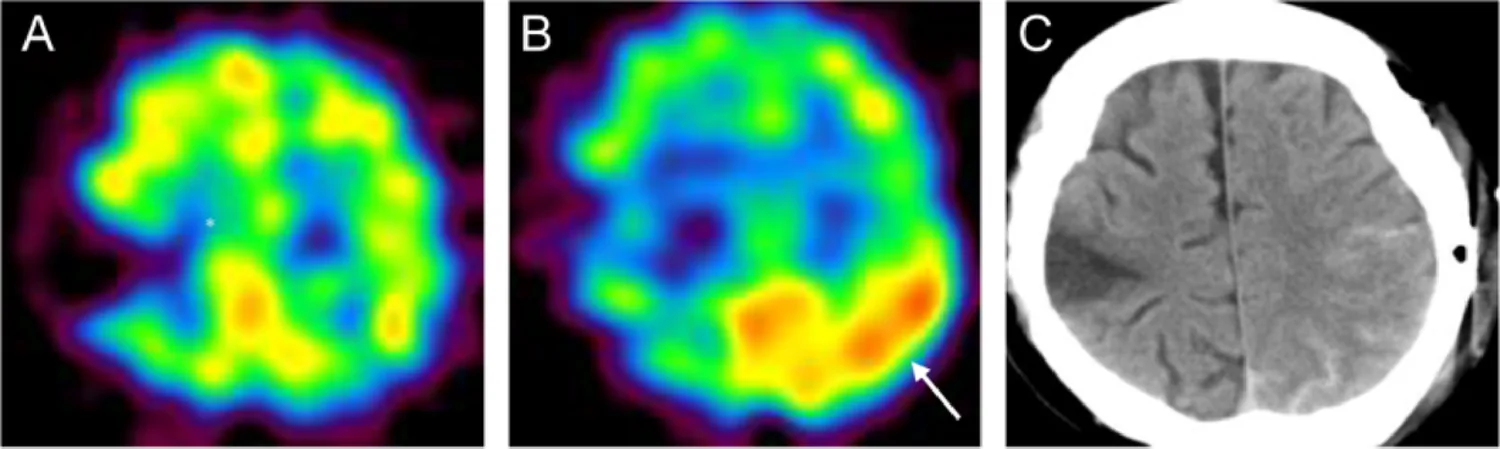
Case 2: Perioperative perfusion changes and postoperative hyperperfusion (Phase 3). A Preoperative 123I-IMP SPECT shows reduced cerebral perfusion in the bilateral middle cerebral artery (MCA) territories. A focal area of markedly decreased uptake in the right hemisphere (asterisk) corresponds to a chronic lesion related to prior hyperperfusion injury after right-sided revascularization. B 123I-IMP SPECT obtained on postoperative day 2 after left-sided superficial temporal artery–middle cerebral artery (STA–MCA) bypass demonstrates focal hyperperfusion in the posterior portion of the left MCA territory (arrow). C Non-contrast computed tomography reveals a localized convexity subarachnoid hemorrhage in the left hemisphere, likely associated with the focal hyperperfusion shown in Panel B.
